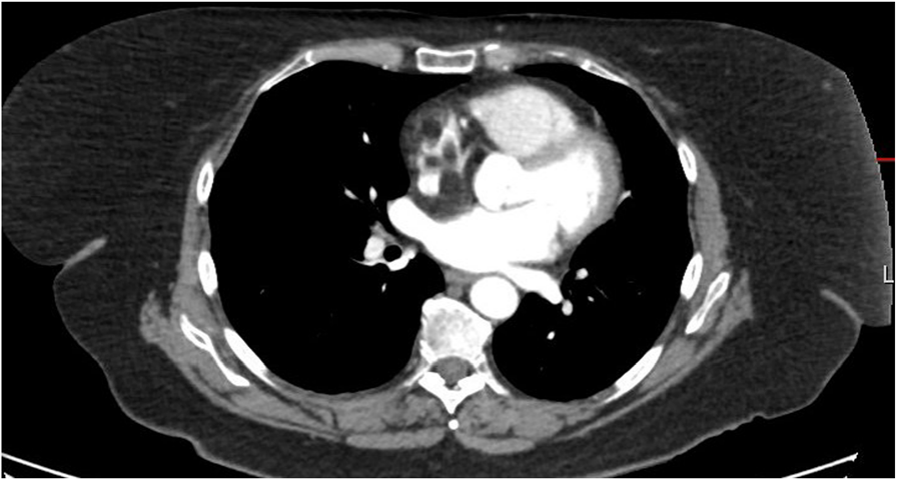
Transverse plane contrast CT thorax showing right atrial mass
